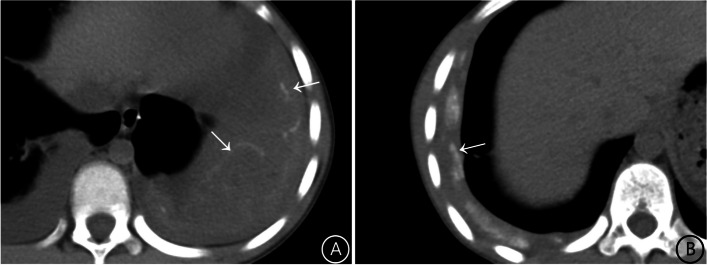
Pleural calcification on CT. A Paragonimiasis, bilateral pleural effusion, linear, map-like calcification of the left pleura (white arrow); B Tuberculous pleurisy, right pleural thickening, right pleural effusion, pleural manifestations of nodular and strip-like calcifications (white arrows)
